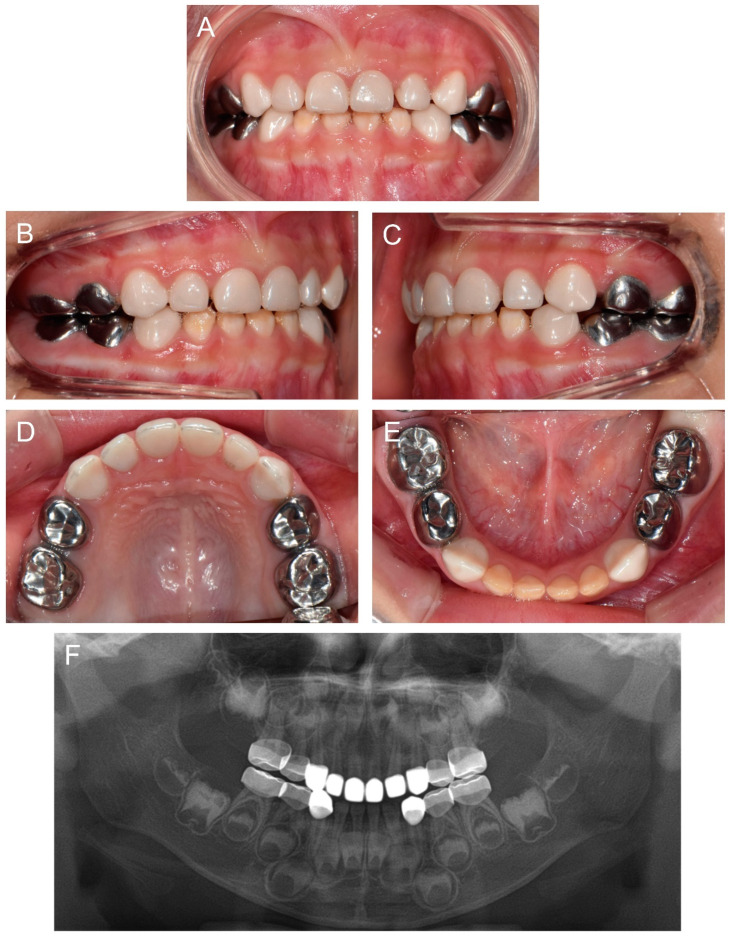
Clinical photos and panoramic radiograph of the proband at age 3 years 11 months. (A–E) Oral health and restorations were well maintained. Anterior open bite was spontaneously corrected with the discontinuance of the finger sucking habit. (F) Panoramic radiograph showed hypocalcified enamel in the developing permanent teeth.
